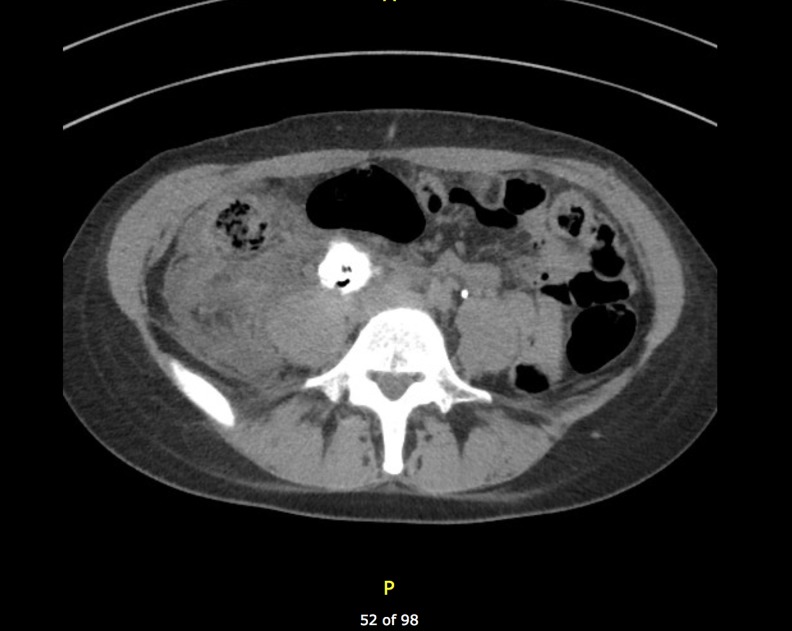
Preoperative CT showing right ureteral granuloma measuring 4.2 × 0.1 × 1.8 cm.
